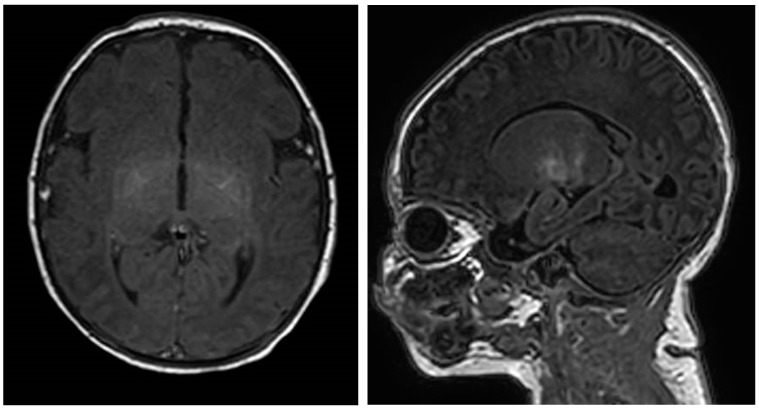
MRI findings in an infant with HINE score >73 and a language delay, Axial and sagittal T1-weighted images show slight increased signal intensity in the lentiform nucleus.
